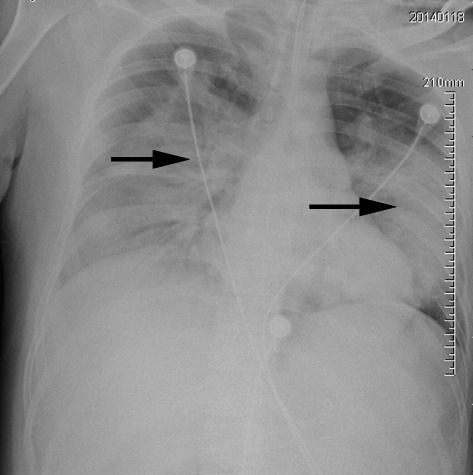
Representative radiographic findings of the laboratory-confirmed 31-year-old Shanghai surgeon infected with H7N9 influenza. Chest radiograph of this patient was taken at 7 days after onset of symptoms, showing bilateral pulmonary infiltrates of airspace consolidation and severe consolidation in the left lobe
